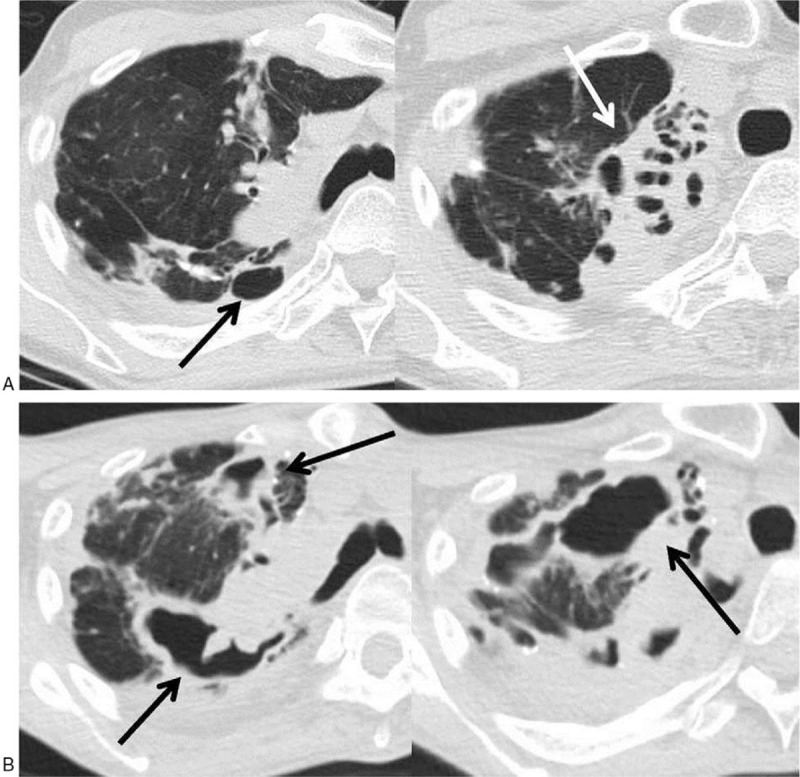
Serial CT scans of *M massiliense* pulmonary disease at time points A and B in a 46-year-old woman. (A) Scans obtained at time point A show bronchiectasis and cavities (arrows) in the right upper lung zone. (B) Scans obtained at time point B (36 months after time point A) show interval progression of cavities (arrows). Total scores (diameter, wall thickness, and extent) for cavitary lesions were 4 (score of 1, 2, and 1, respectively) for time point A and 7 (scores of 3, 3, and 1, respectively) for time point B. CT = computed tomographic.
